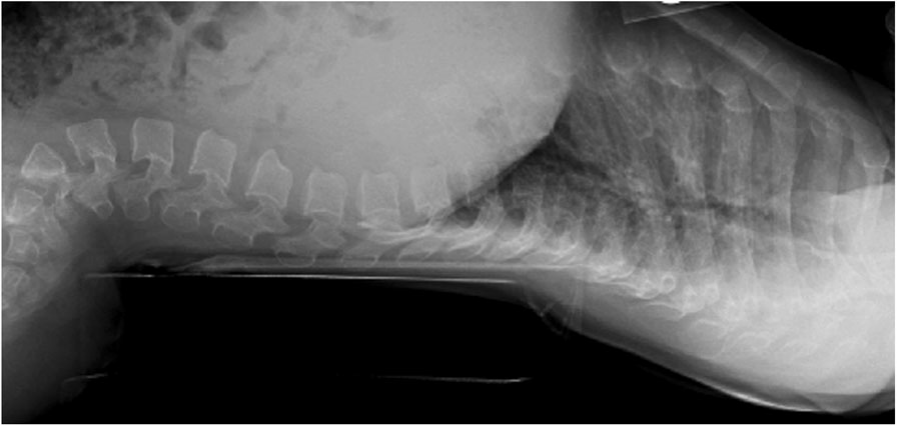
Cross table supine over-a-bolster lateral radiograph shows a mild irreversible kyphotic curve and mild loss of anterior substance of two vertebrae. This view or, alternatively, a cross table prone lateral radiograph, can be used to assess the irreversible component of kyphotic curvature
